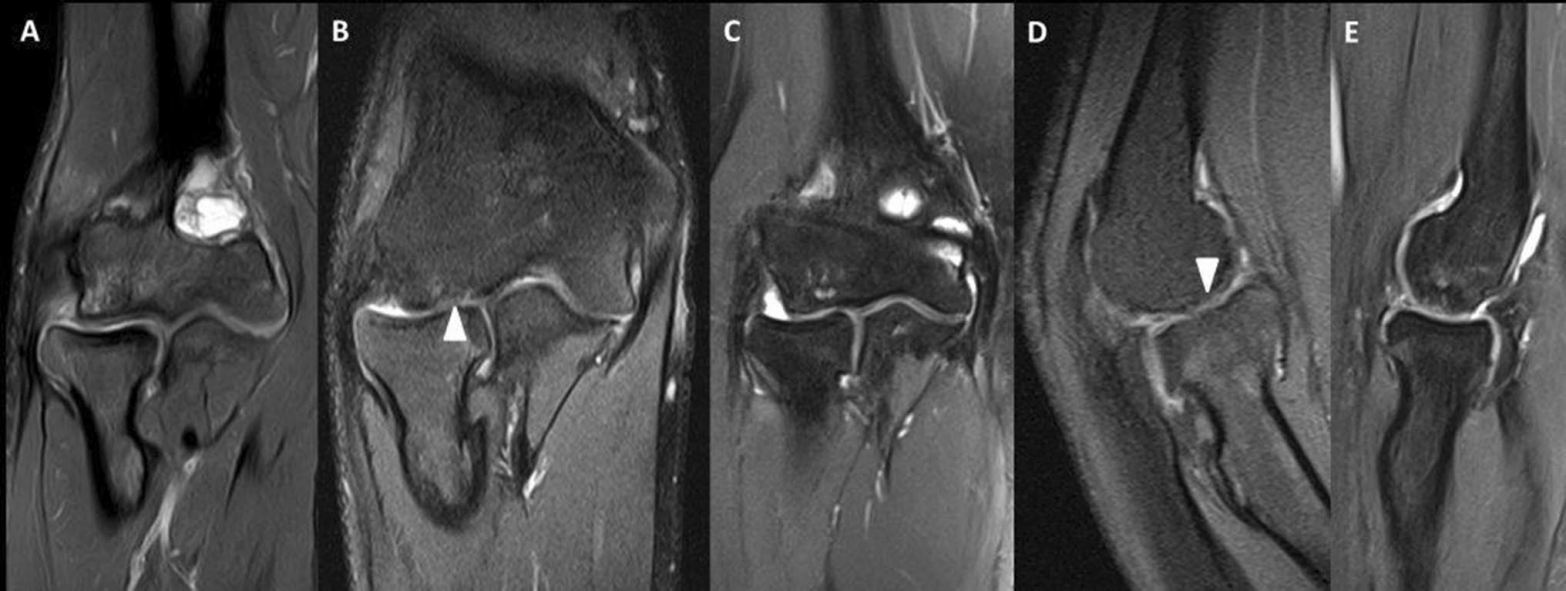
The postoperative MRI sequences of cases 4 (a), 5 (b, c) and 2 (d, e) are shown. Reduction of OCD-related edema is visible in all cases. Fibrous regenerate cartilage is highlighted in B and D (*arrowhead*)
